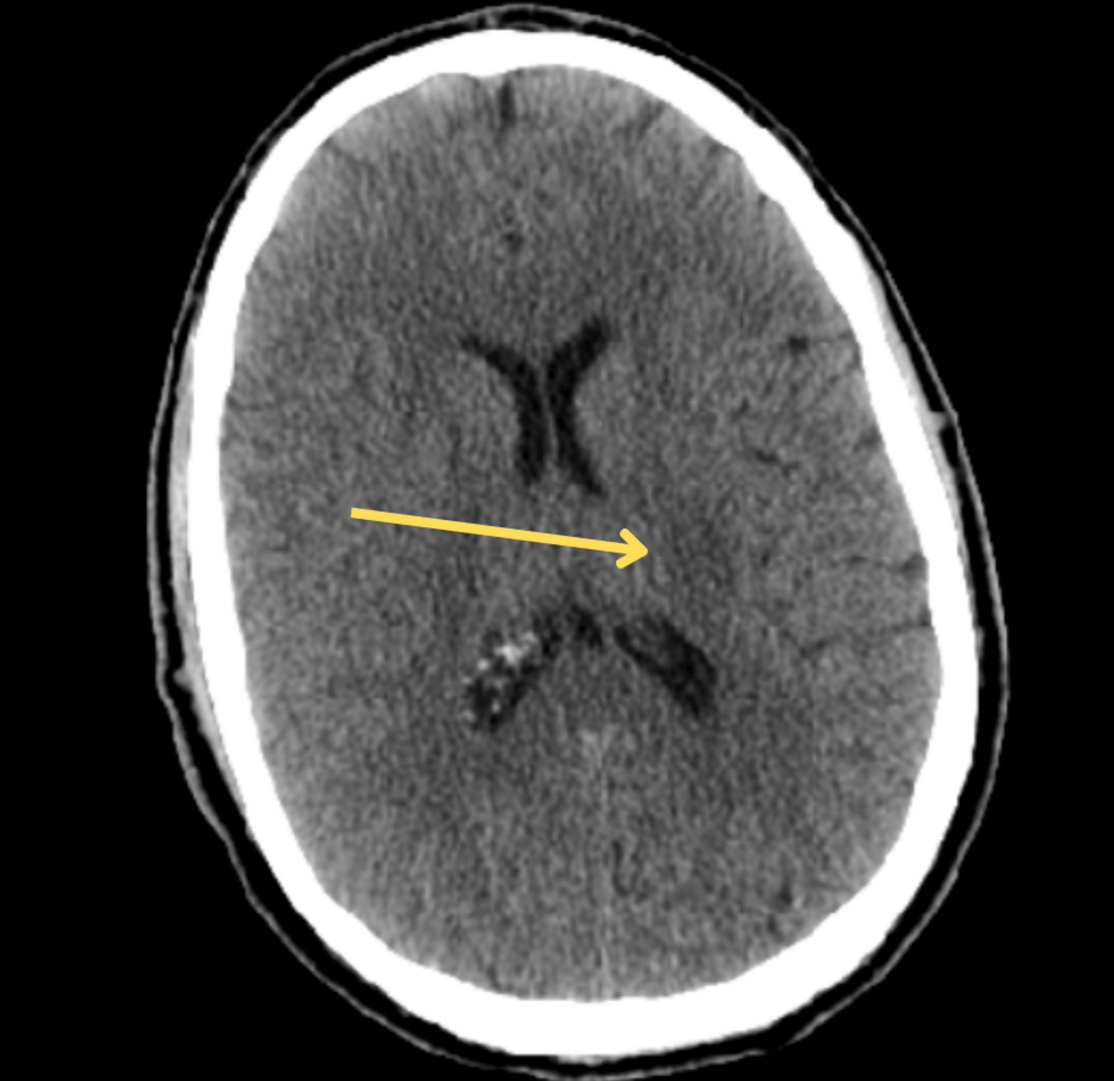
CT head showing a small area of new low attenuation in the left temporal lobe adjacent to the ventricle, raising suspicion for acute infarction
